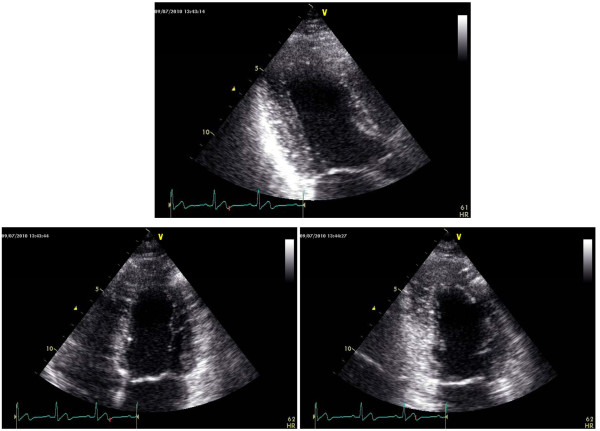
2-D apical views at end-systole (upper panel: apical long-axis view, lower panels: apical 4-chamber view on the left, 2-chamber view on the right) for subsequent STE or AFI analysis.
